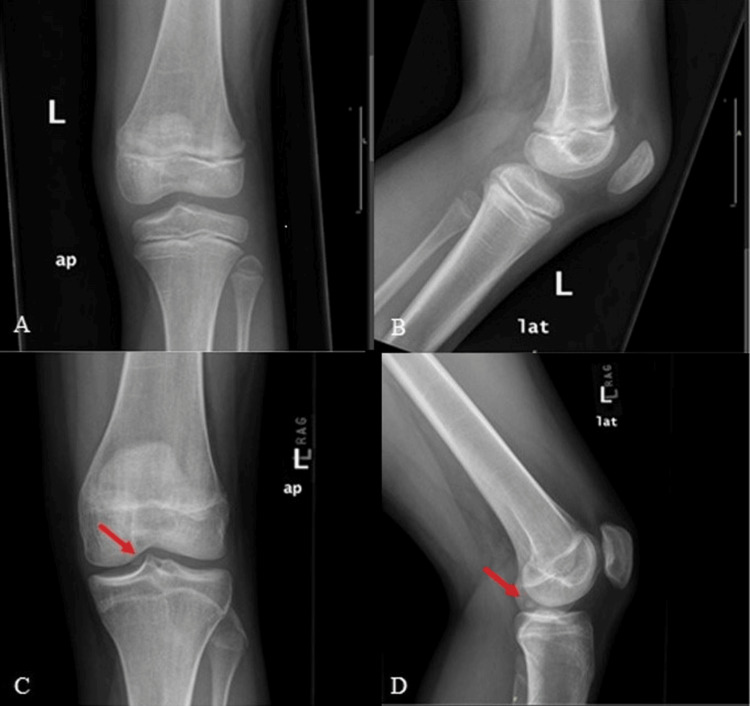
Radiographs of the left knee at nine years of age showing no evidence of a meniscal ossicle on both anterior-posterior (A) and lateral (B) views. Radiographs of the left knee at 16 years of age suggestive of a bony ossicle (arrow) in the posterior portion of the medial compartment on both anterior-posterior (C) and lateral (D) views.
